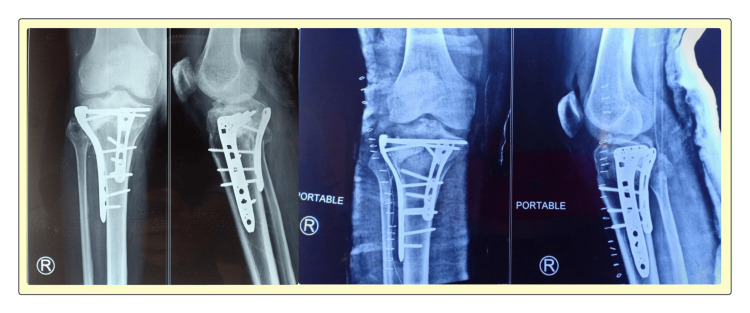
X-ray images show successful internal fixation using locking compression plates and screws, ensuring fracture stabilization and alignment. These images help assess implant positioning, bone healing progression, and potential complications during the recovery period.
